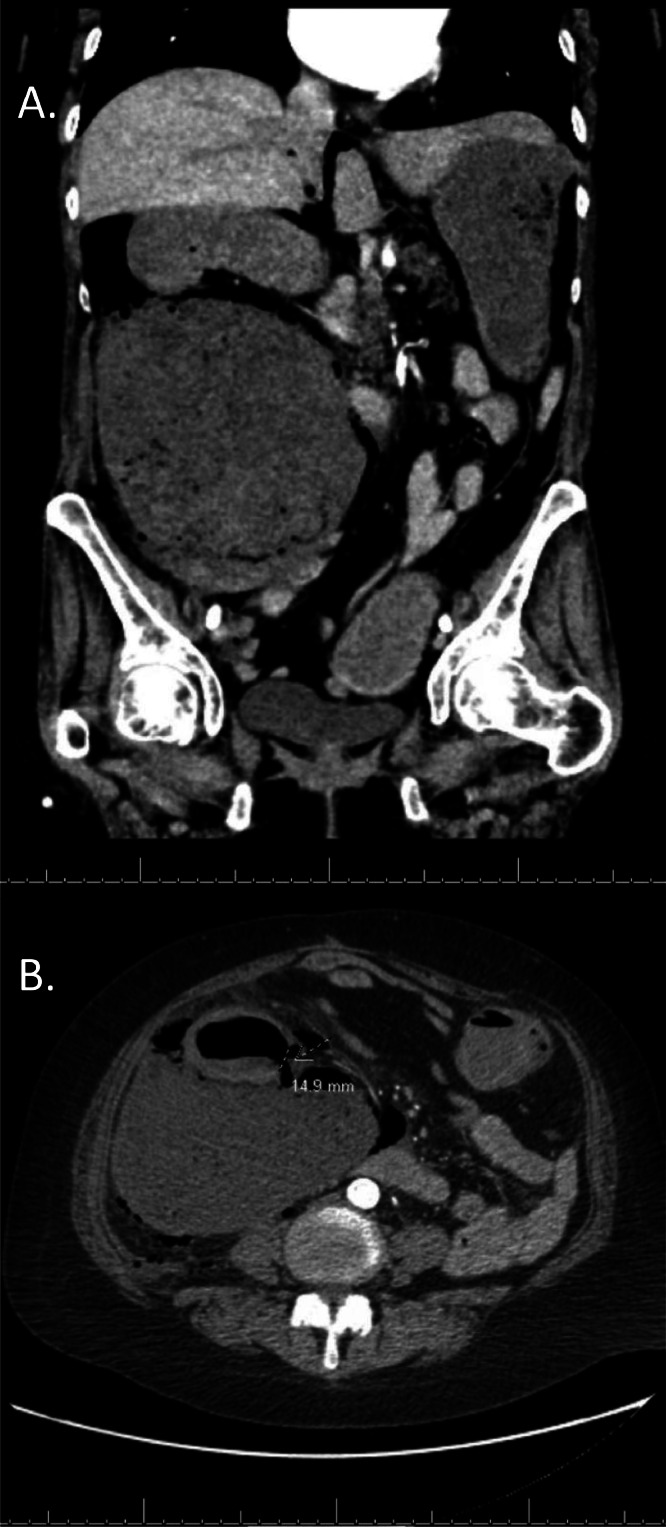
(A) Coronal CT image shows atypical collection of air in the right upper quadrant and (B) axial CT image reveals perforation at the posterior medial wall of the proximal ascending colon.
